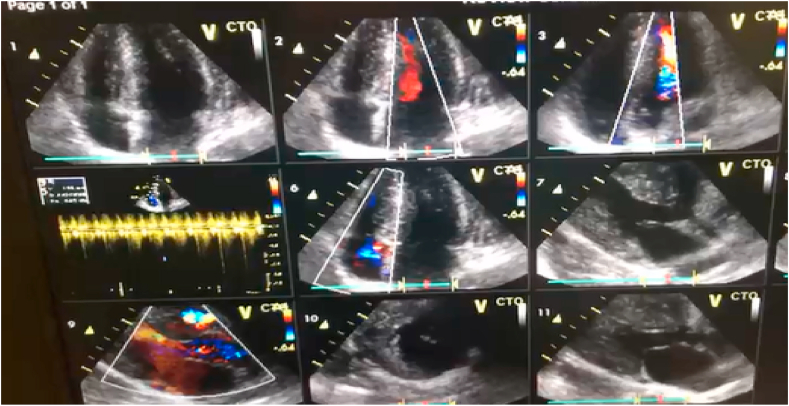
First echocardiography of patient in hospital.
